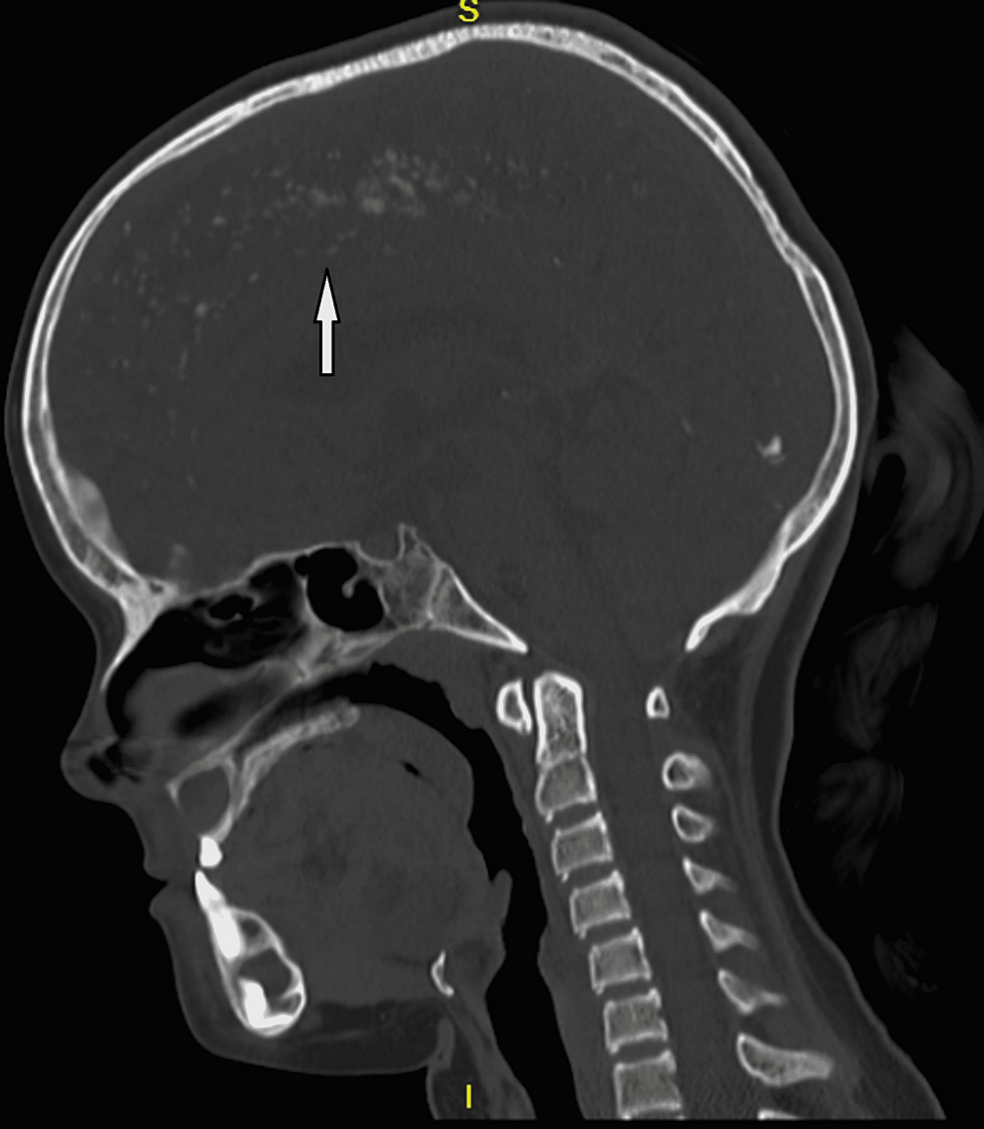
CT shows calcification in falx cerebri and tentorium cerebellum
